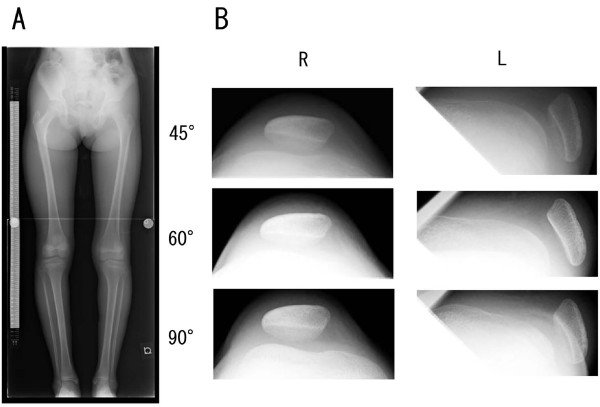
Bilateral X-rays of the lower extremities, including skyline view. The modified Q-angle is 36° (Fig. 2-A). The skyline view demonstrates that the patella is dislocated at 45°, 60°, and 90° of flexion in contrast to the contralateral side, which is consistently in position at the femoral trochlea (Fig. 2-B).
